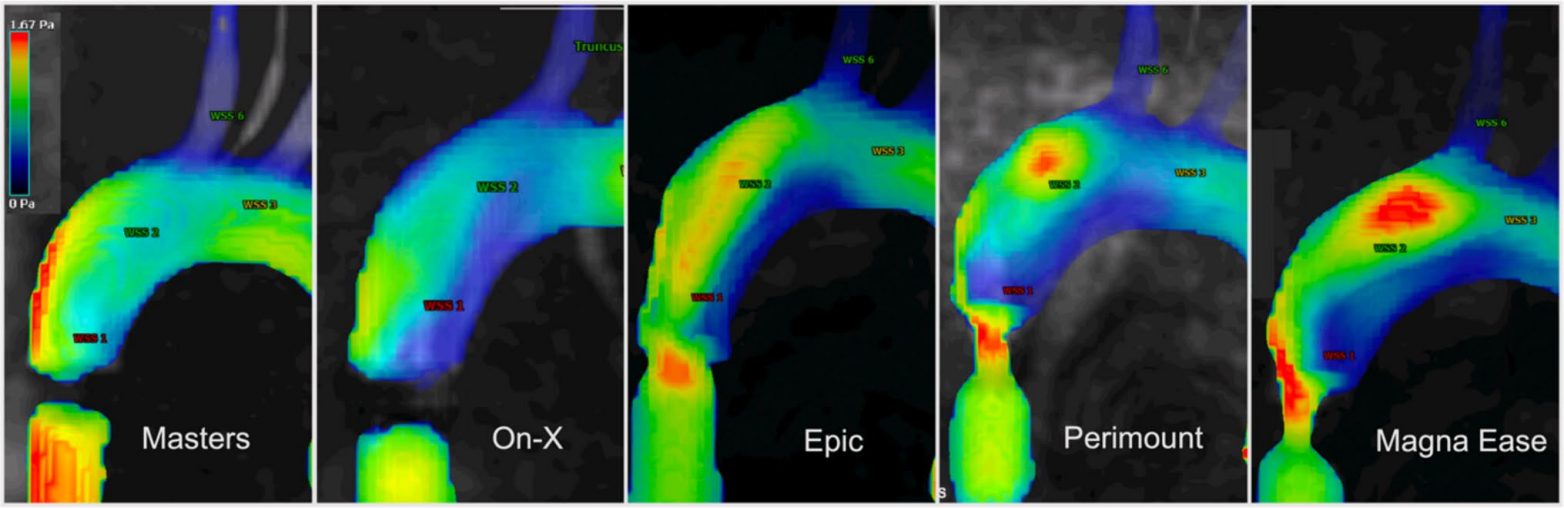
4D-MRI wall shear stress visualization for all surgical valves during peak systole; Mechanical valves showed artefacts around the valvular plane, due to the valve material
